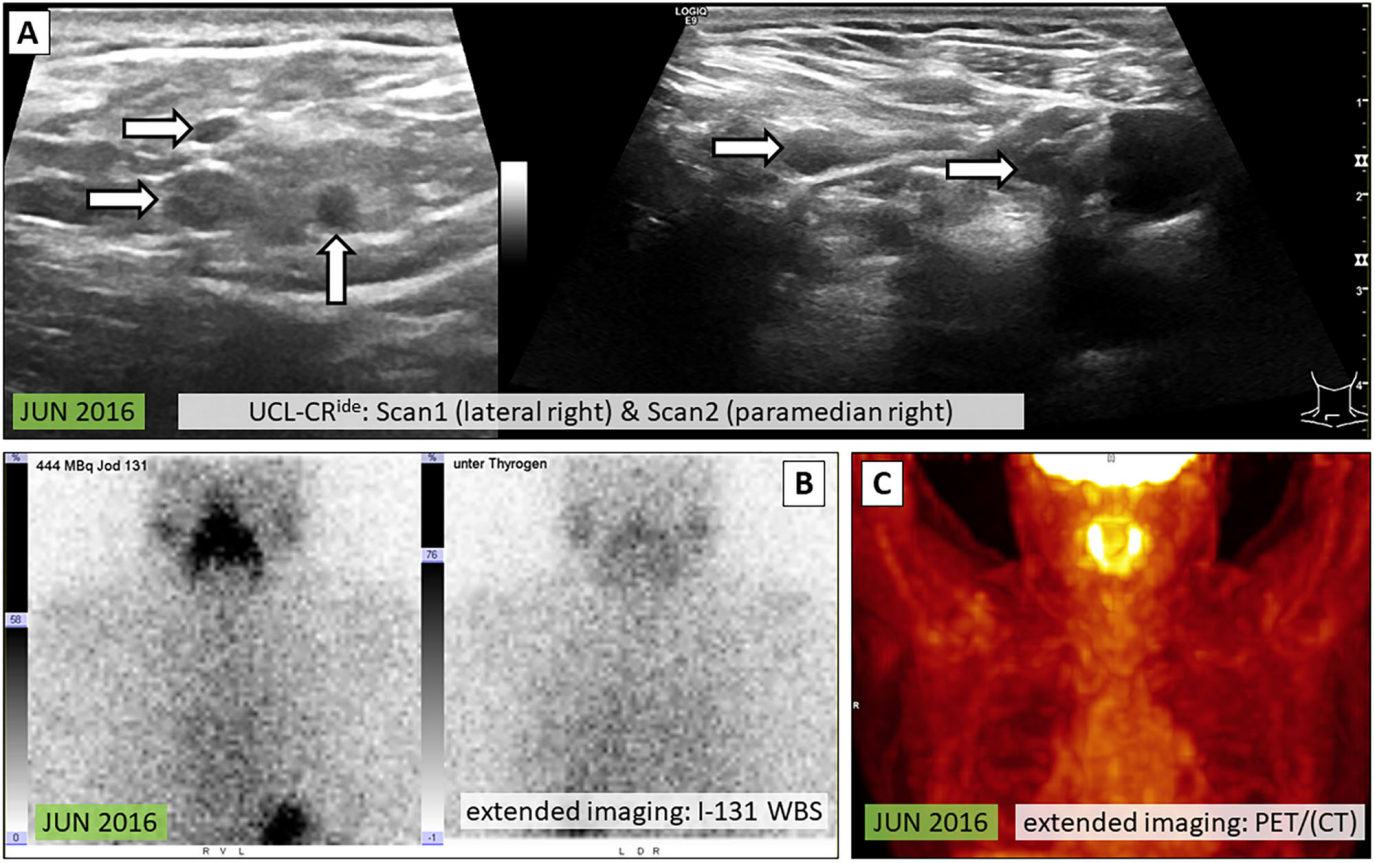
Patient case II: UCL-CR^ide^ without confirmed diagnosis / false diagnosis. 64-year-old female at initial diagnosis 2008 (PTC pT1b pN1b cM0). The PACS review of the UCL revealed multiple suspicious lymph nodes supraclavicular right [A, white arrows], measuring up to 7 mm, that have not been identified on conventional live US during regular FU. TG was not measurable. Additional extended imaging included I-131 WBS [B] and F-18-FDG PET/CT [C] did not show any suspicious findings. No PTC relapse occurred in further FU. Thus, the diagnosis of the UCL-CR could not be confirmed. UCL-CR^ide^ standardized cervical ultrasound cine loops with clinical relevance—identification of a suspicious finding that was not identified on conventional live ultrasound, PTC papillary thyroid carcinoma, PACS Picture Archiving and Communication System, US ultrasound, FU follow-up, TG thyroglobulin, I-131 WBS iodine-131 whole body scintigraphy, F-18-FDG PET/CT fluorine-18-fluorodeoxyglucose positron emission tomography computed tomography
